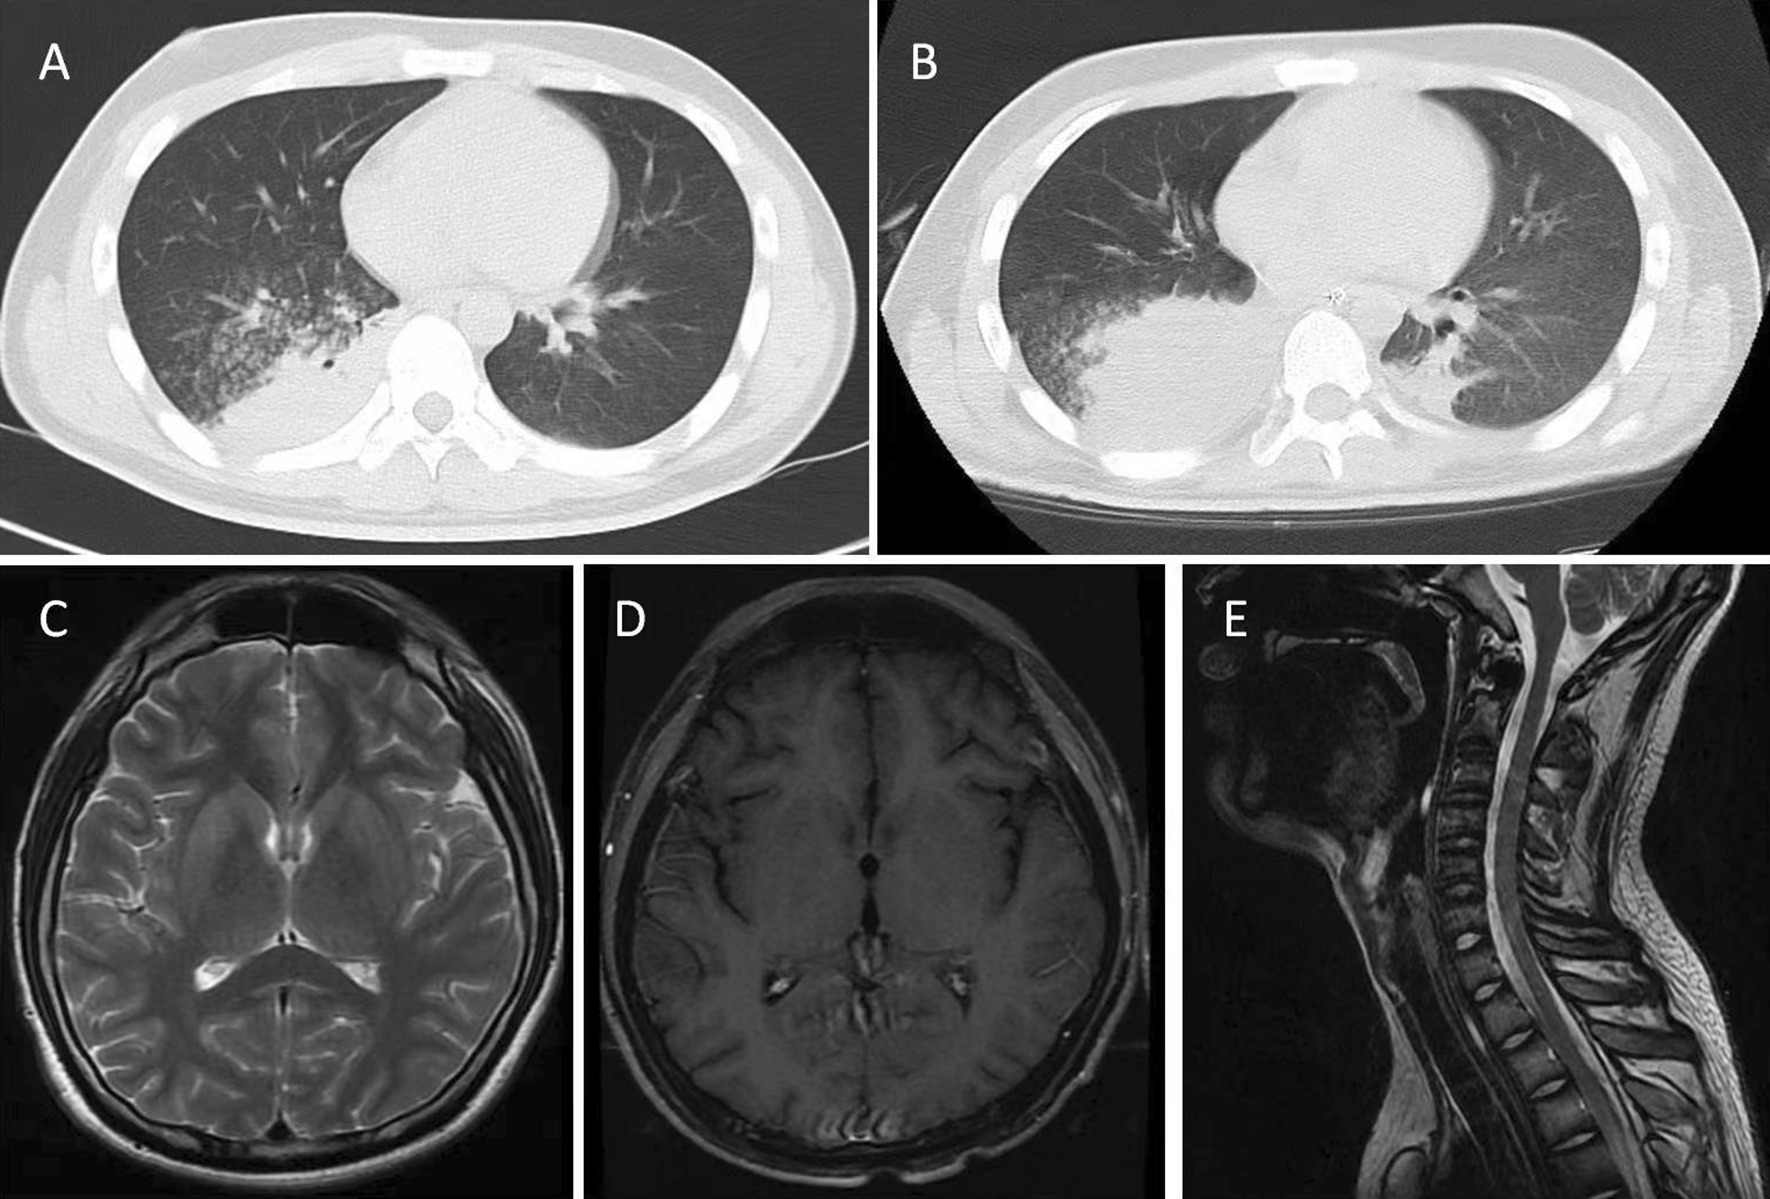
The radiographs in this case. A At 19th day of illness onset, lung CT indicated consolidation in the right lung. B At 24th day of illness onset, lung CT indicated aggravated consolidation in the lower lobe of bilateral lung adjacent to the pleura, mainly in the right lung. C At 5th day of illness onset, brain MRI (T2 weighted image) was normal. D At 24th day of illness onset, enhanced brain MRI was normal. E At 24th day of illness onset, spine MRI (T2 weighted image) was normal
